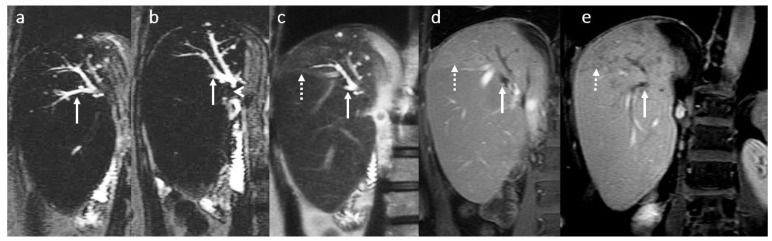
Focal intrahepatic acute cholangitis in a female patient, 70 years old, 2 years after LDLT (right liver lobe from her daughter) with jaundice and fever. MRI evaluation in coronal 2D T2 SSFSE long TE (a,b) and short TE acquisition (c) in association with coronal 3D T1 FS after gadoxetic acid i.v. injection in arterio-portal phase (d) and transitional phase (e): moderate dilatation of posterior right intrahepatic bile ducts (white arrow) secondary to a tight fibrotic stenosis of the bilio-digestive anastomosis (head arrow) involving the posterior right biliary duct ramifications; mild T2 hyperintensity and enhancement of the adjacent liver parenchyma visible in late arterial phase in association with hypointensity in the transitional phase are suggestive for inflammatory changes (dotted arrow).
